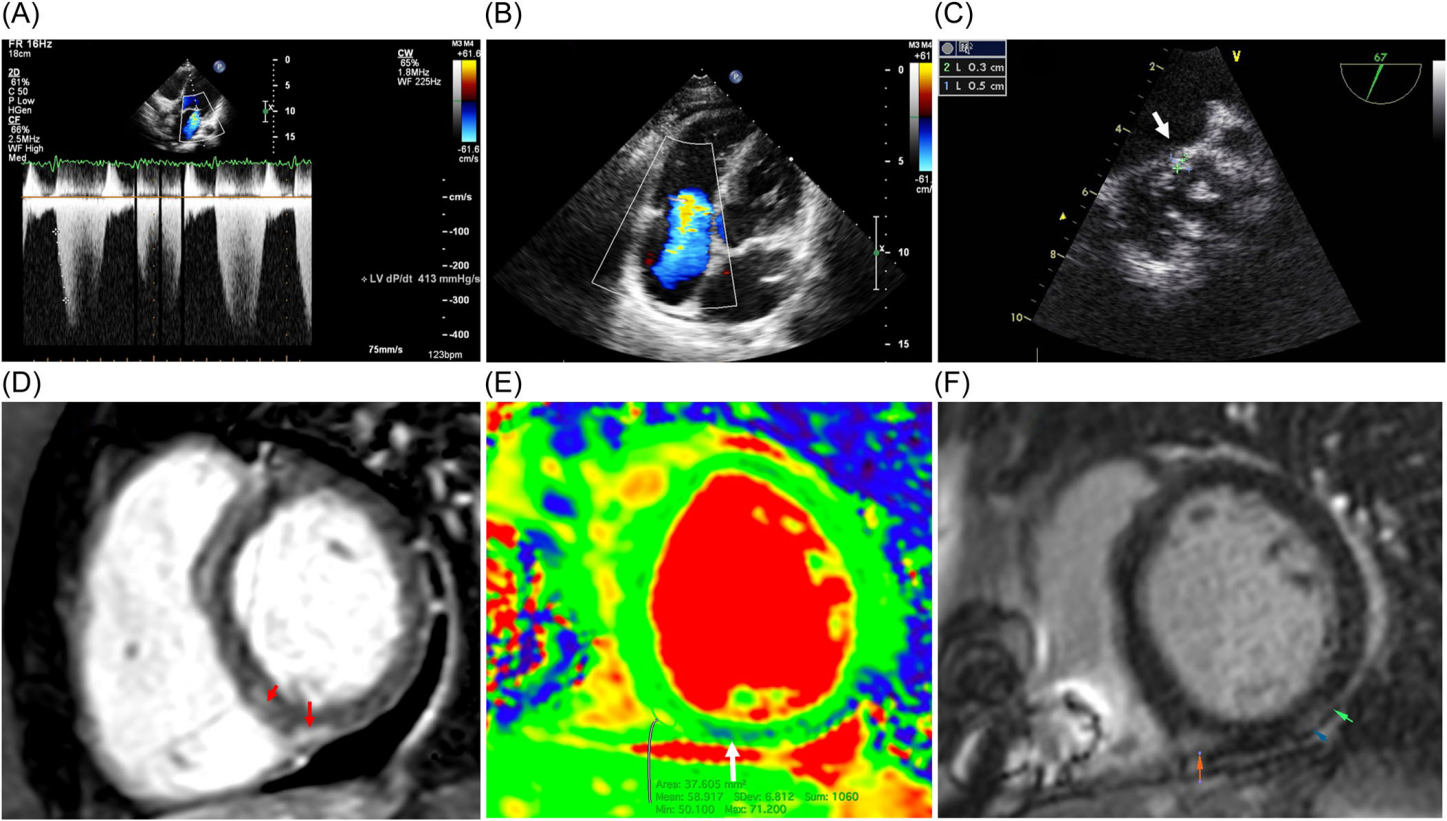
Echocardiography and magnetic resonance imaging in patients with post‐COVID myocarditis. Upper series—echocardiography: (A) decreased *dp*/*dt* (413 mmHg); (B) severe tricuspid regurgitation due to dilatation of the right ventricle; (C) vegetation on the bicuspid aortic valve measuring 3 × 5 mm (arrow), transesophageal study. Lower series—MRI: (D, F) late gadolinium enhancement in the posterior septal and posterior segments of the left ventricle (arrows); (E) edema along the posterior septal segment of the left ventricle (T2 map).
